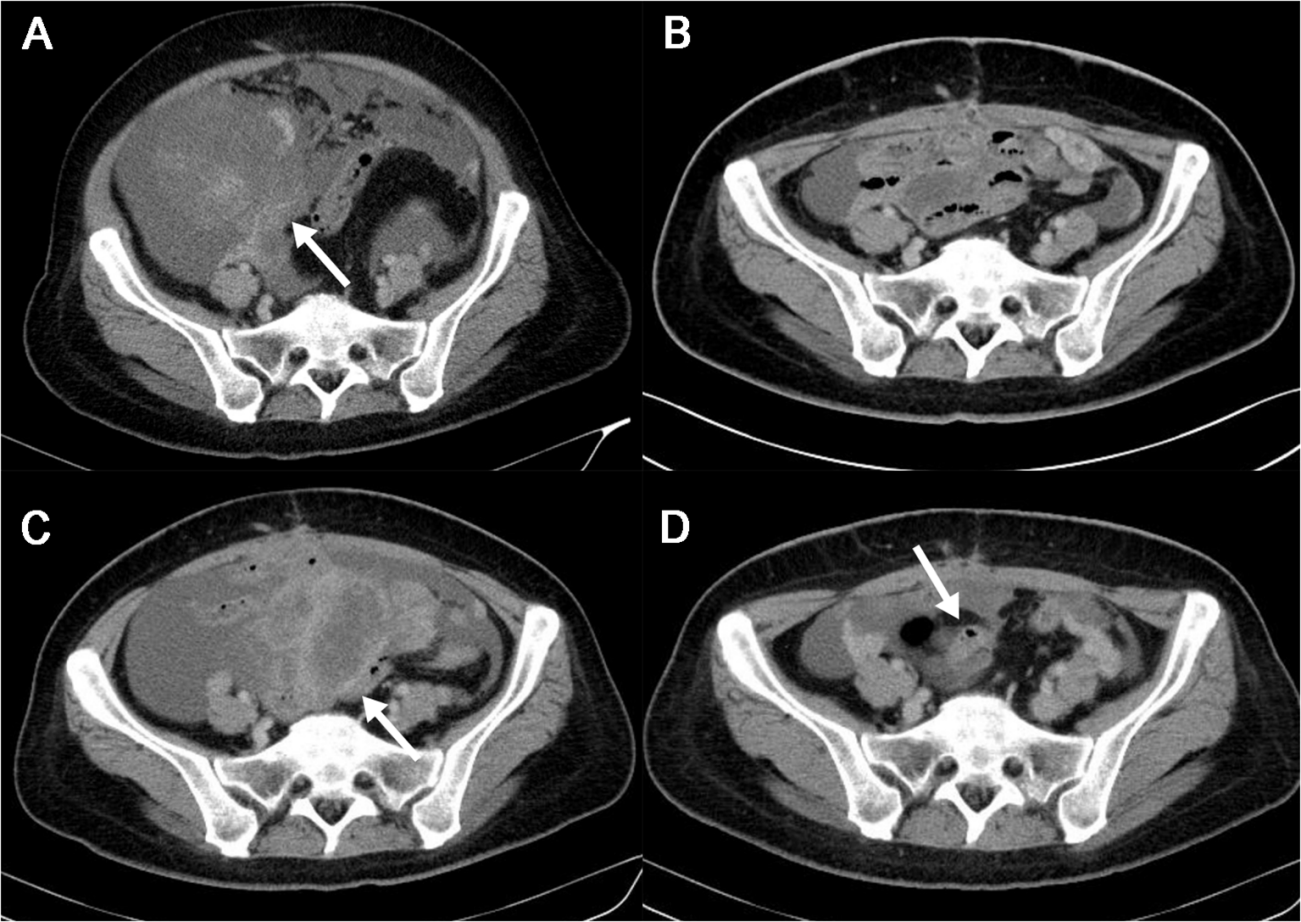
The patient’s imaging features during whole course of the disease in abdominal and pelvic enhanced CT. (A). Preoperative Abdominal and Pelvic Enhanced CT in June, 2023. Multiple soft tissue masses with blurred edge were observed in the abdominal cavity(white arrow). After contrast enhancement, the masses showed obvious uneven enhancement, the largest one was about 19.5*16.8cm. Massive ascites and multiple retroperitoneal enlarged lymph nodes were seen in the abdomen and pelvis. (B). Postoperative Abdominal and Pelvic Enhanced CT in August, 2023. Ascites was still in the pelvic cavity. (C). Abdominal and Pelvic Enhanced CT in September, 2023. Interim assessment of chemotherapy show disease progression(tumors recurred as shown by white arrow). (D). Abdominal and Pelvic Enhanced CT in November, 2023. One month after initiating ALKi treatment, assessment showed significant tumor shrinkage and reduction in ascites(white arrow). ALKi, anaplastic lymphoma kinase inhibitor.
